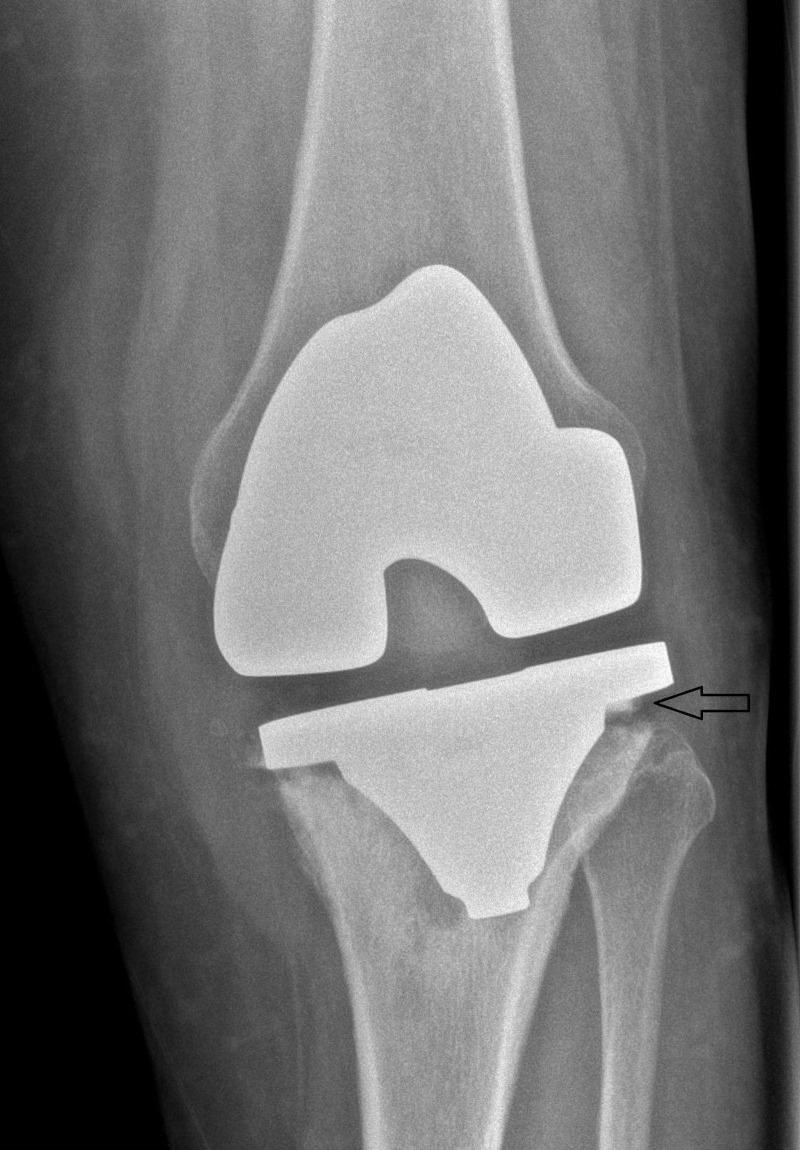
Preoperative X-ray of the Left Knee (Anteroposterior View) Showing Loosening of Tibial Component
